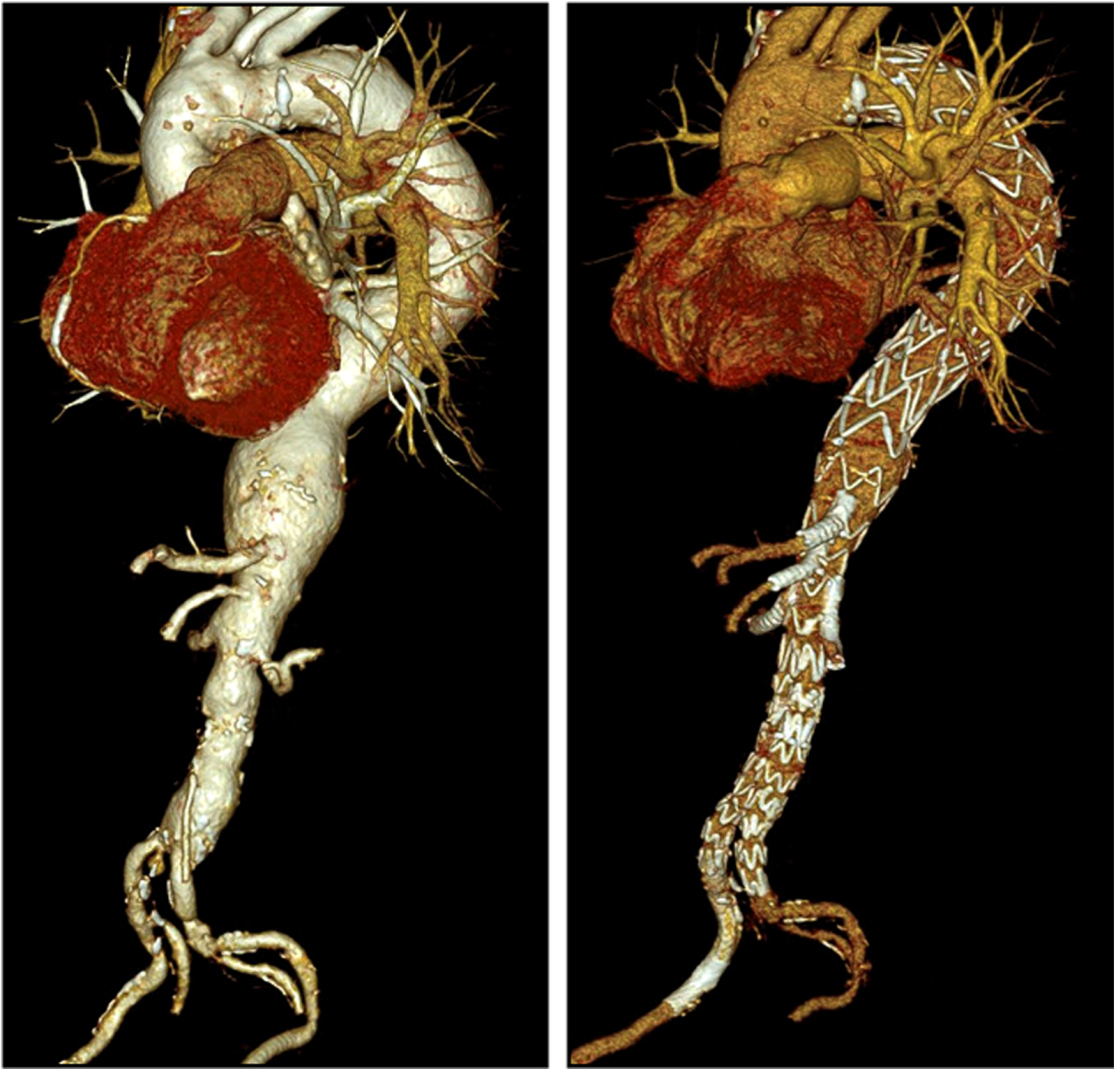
Pre- and postoperative CTA in 3D volume rendering technique. Left: preoperative TAAA type II. Right: postoperative after iBEVAR. CTA, computed tomography angiography; TAAA, thoracoabdominal aortic aneurysm.
